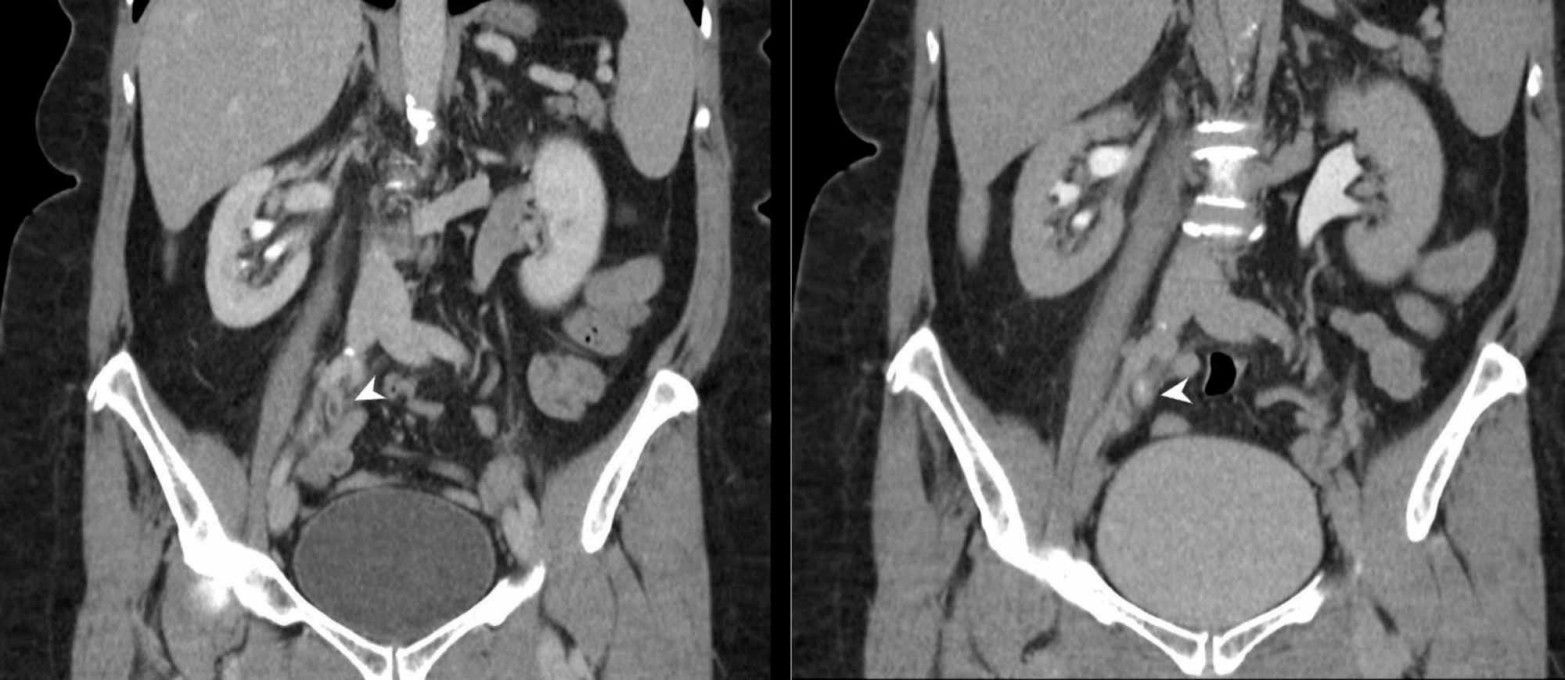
Ureteric TCC (CTU) CT urography (coronal reformats) demonstrates a 4-cm segment of circumferential urothelial thickening at the junction of the mid- and distal-right ureter (arrow heads) with abnormal urothelial enhancement. There is secondary obstruction of the right collecting system with the transition point noted at the level of abnormal urothelial thickening which is highly suspicious for a right-sided urothelial TCC. TCC: Transitional cell carcinoma
